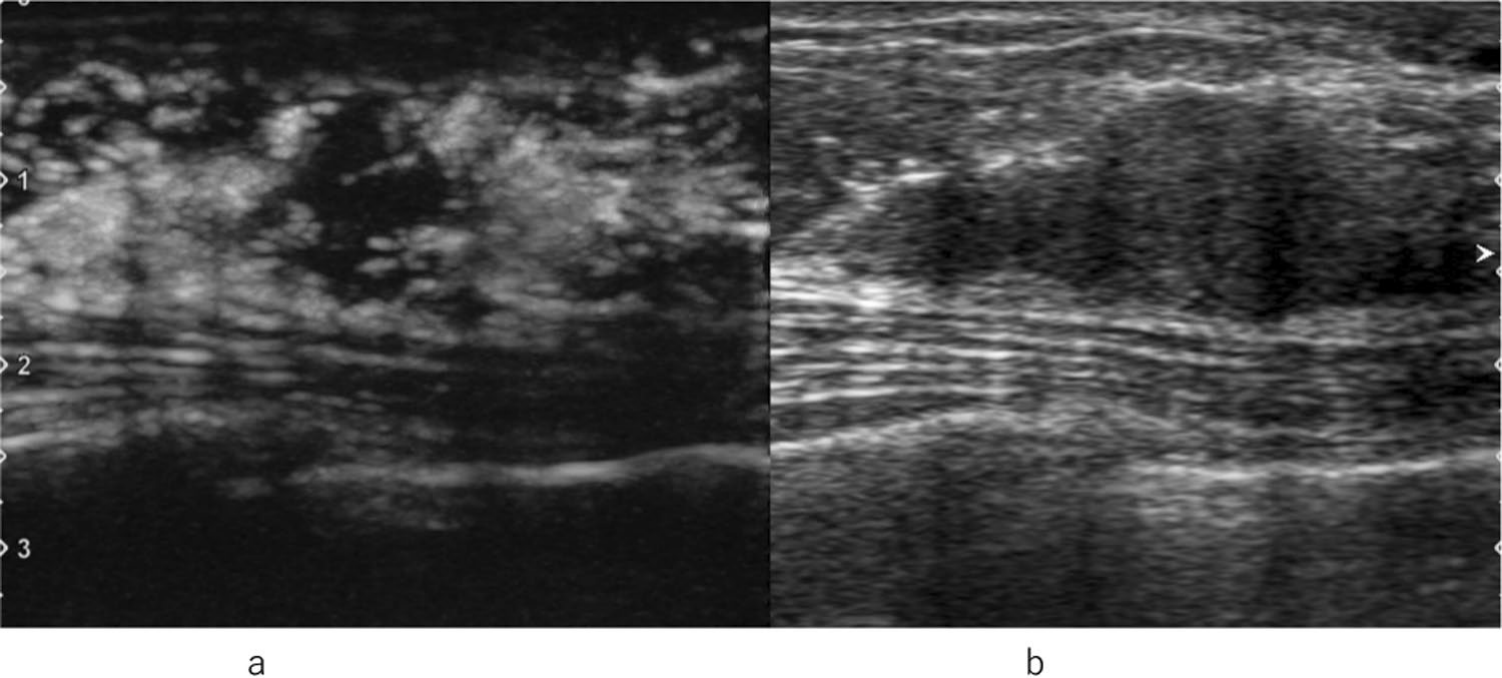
Invasive ductal carcinoma. a Contrast-enhanced ultrasound shows heterogeneous enhancement with a clear defect. b Conventional ultrasound shows a hypoechoic area
